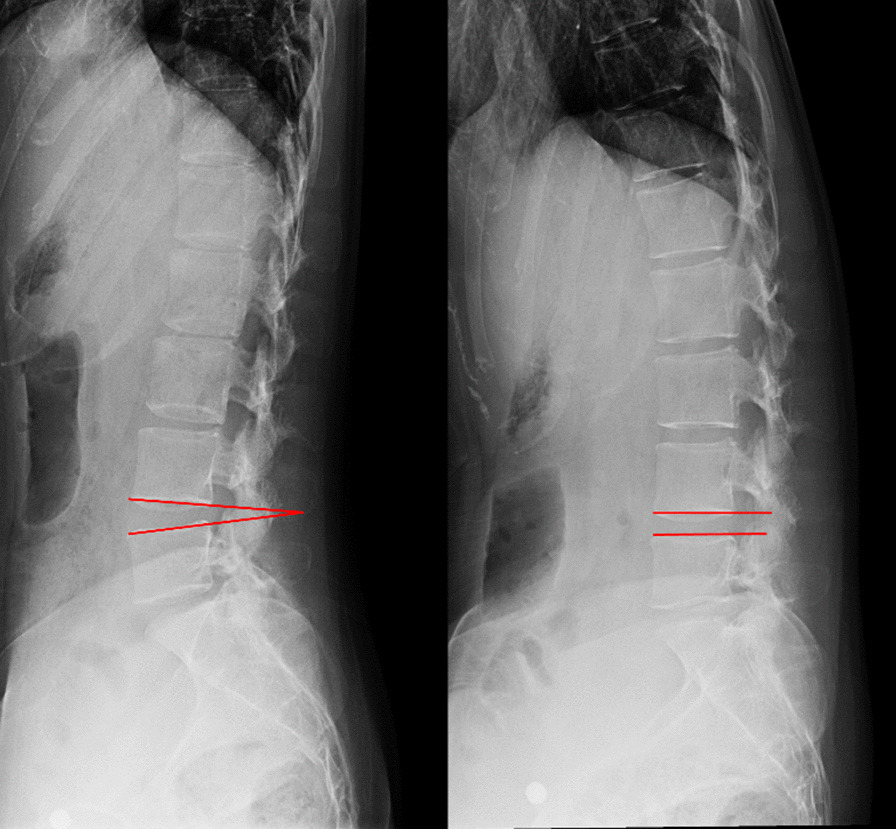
The measurement of intervertebral angle on flexion–extension radiographs
